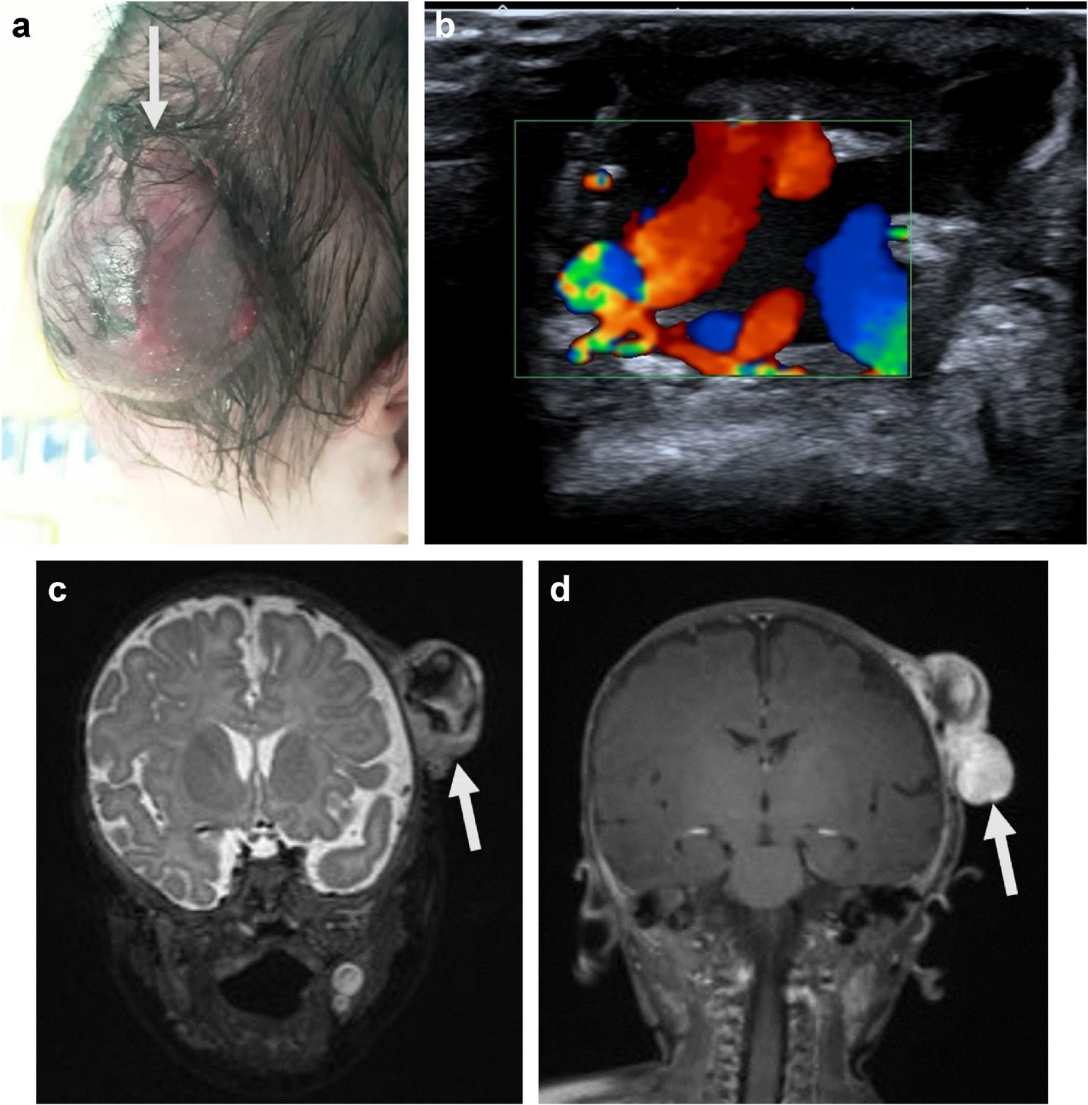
Congenital sarcoma in a boy at 4 months of age. a A solid purple lesion was noted in the scalp at birth (*arrow*). It was initially diagnosed as a congenital hemangioma. However, due to continuous growth in the first months of life, the diagnosis was reconsidered. b Oblique Doppler ultrasound image shows turbulent (multicolor) high flow within the mass. c Coronal T2-weighted magnetic resonance image shows inhomogeneous signal intensity within the lesion. d Postcontrast coronal T1-weighted magnetic resonance image shows enhancement of the lesion extending to the surrounding soft-tissues, which is not typical of a congenital hemangioma. Biopsy confirmed spindle cell sarcoma
